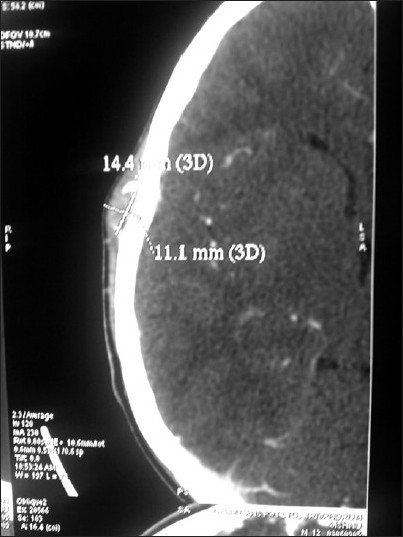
Contrast CT scan, axial section, shows homogeneous enhancement of the lesion located in the right high temporal region. Note the intact inner table and interruption of the outer table of the skull
